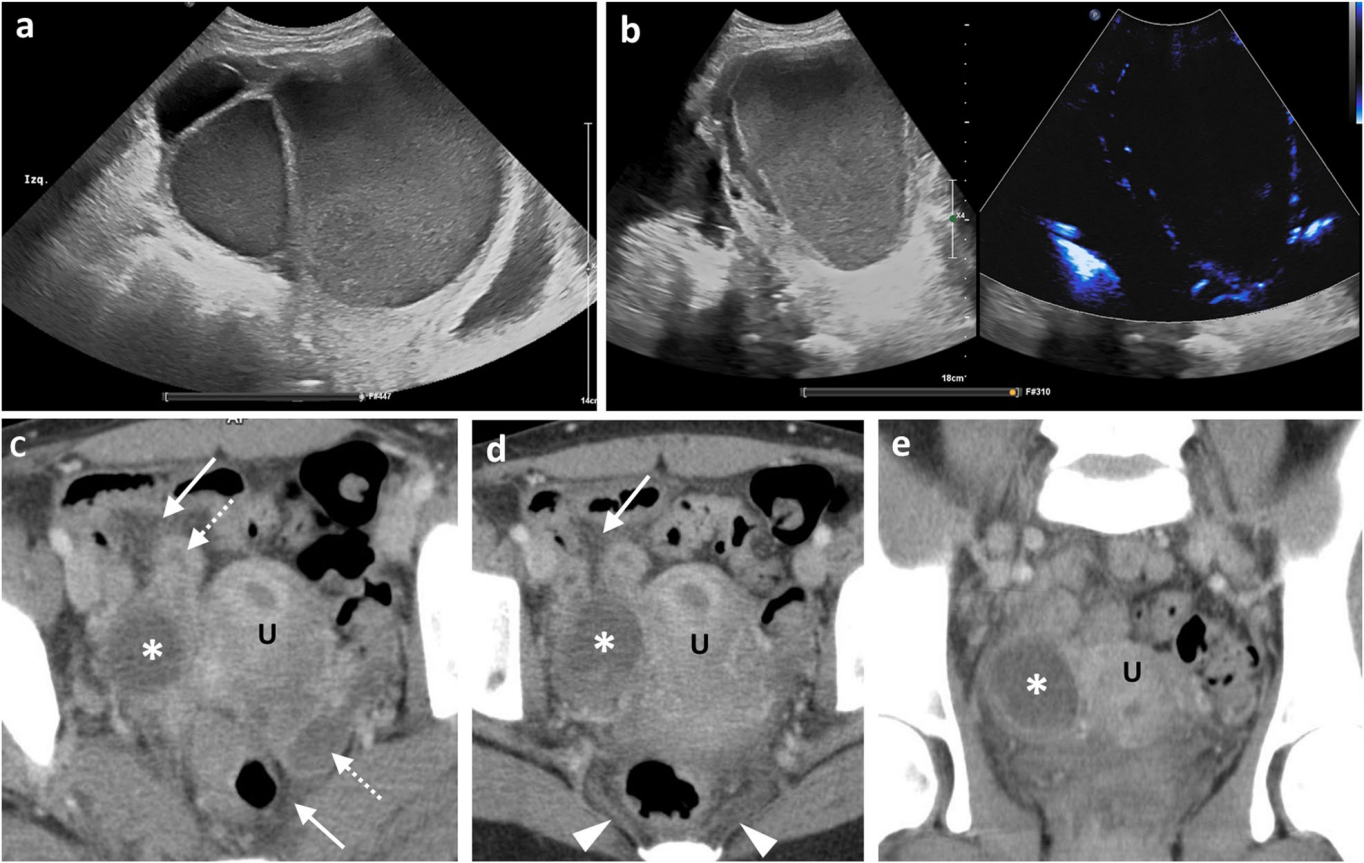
Surgically confirmed tubo-ovarian abscess in two different patients. a, b Middle-aged woman with lower abdominal pain, fever and vaginal discharge. a Transabdominal grey-scale ultrasound shows a large cystic complex mass representing a tubo-ovarian complex with echogenic content consistent with pus. b Microflow ultrasound imaging demonstrates peripheral vascularity in the walls of the cystic lesion without internal vascularity in keeping with the cystic nature of the mass. c–e Young woman with pelvic pain and raised inflammatory markers. Consecutive axial (a, b) and coronal (c) CECT images, venous phase, show bilateral pyosalpinx (dotted arrows) and a right ovarian abscess (asterisk). Note the fat stranding around the fallopian tubes (arrows) and the markedly thickened uterosacral ligaments (arrowheads)
